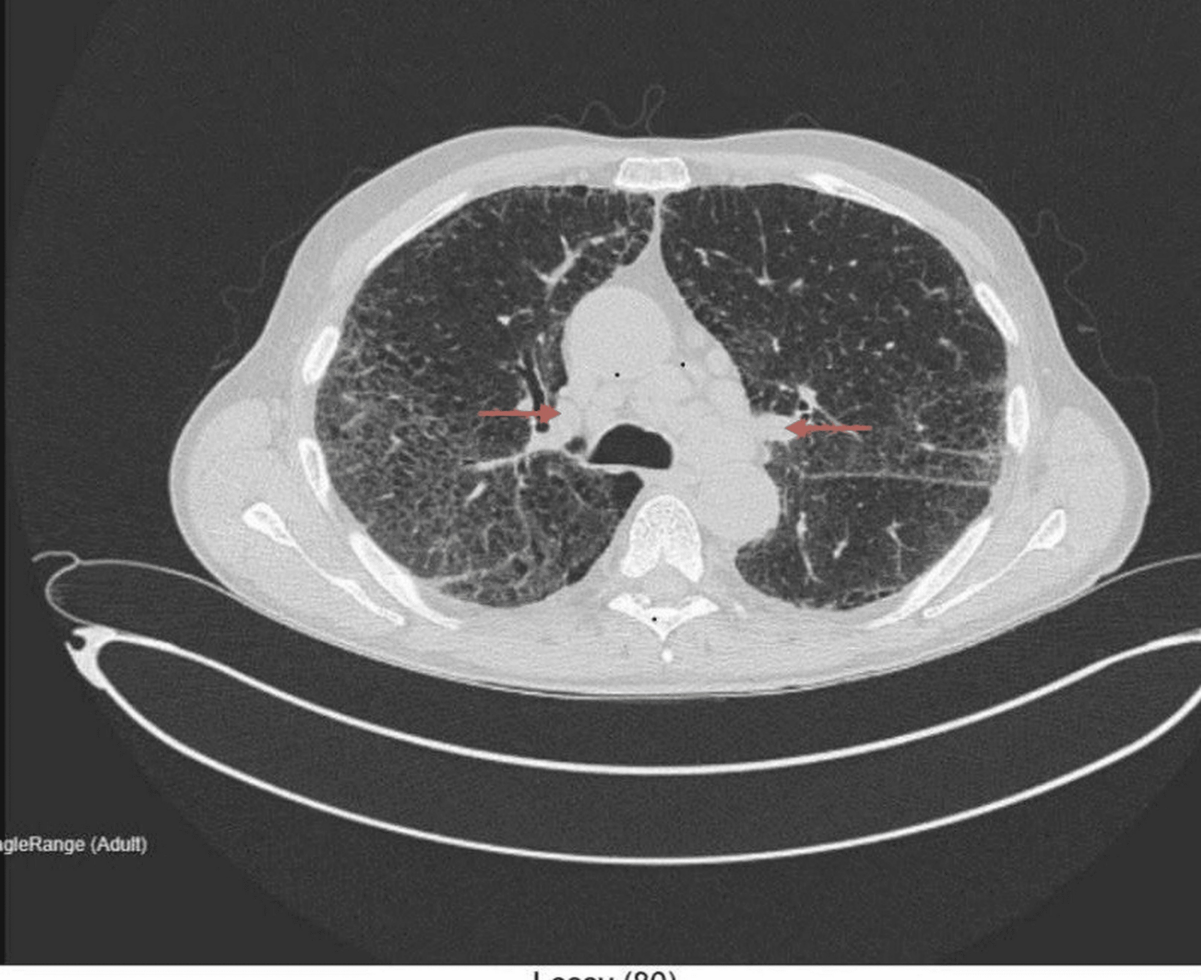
Axial non-contrast CT thorax, abdomen, and pelvis (lung window) Technique: Non-contrast computed tomography (CT). Findings: Widespread ground-glass changes with fibrotic interstitial lung alterations and multiple enlarged mediastinal and bilateral hilar lymph nodes are noted (red arrows). No pleural or pericardial effusion. Abdominal organs unremarkable, no lymphadenopathy or bone lesions. Conclusion: Fibrotic interstitial lung changes with indeterminate mediastinal hilar lymph nodes. No radiologic evidence of malignancy.
